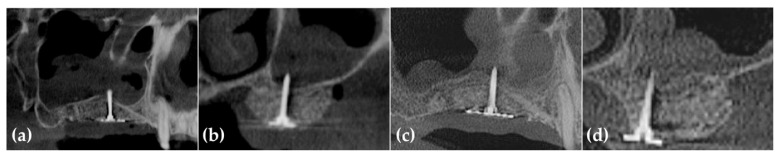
(a) Post-operative panoramic image of CBCT shows vertically grafted bone; (b) Cross-sectional images of CBCT reveal horizontally grafted bone; (c) Panoramic image of CBCT after 6 months of healing demonstrates that vertical alveolar ridge augmentation is distinctly observed; (d) The cross-sectional image at 6 months post-surgery demonstrates that horizontal ridge augmentation was remarkably well achieved.
